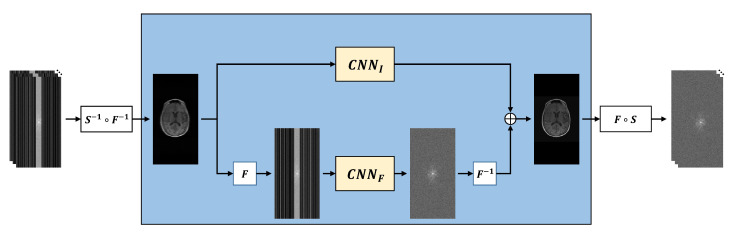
CNN-based regularization block. An MR image is reconstructed in parallel in the image domain and the k-space domain with two U-Nets and then added.
